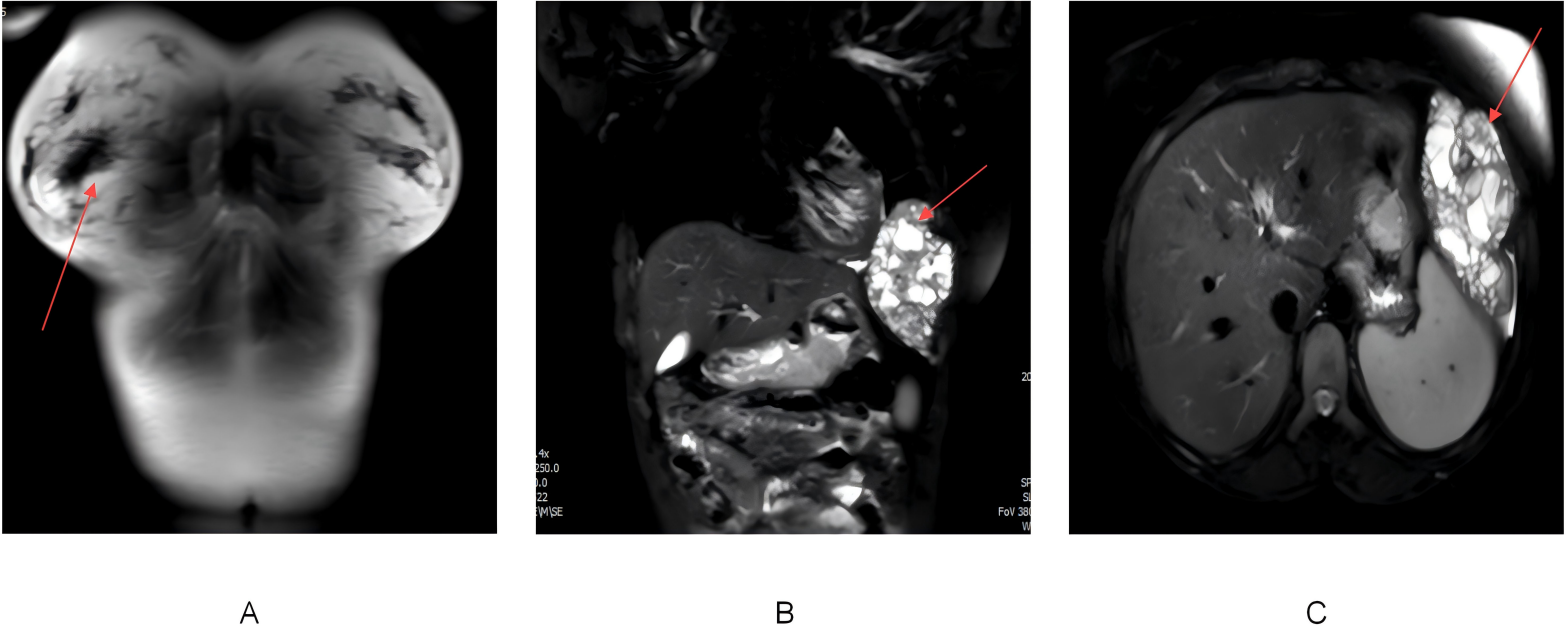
(A) MRI of the back and chest soft tissues showing expansile destruction of the left 6th rib, with an abnormal soft tissue mass in the adjacent pleural cavity. The mass appears heterogeneous on T1WI and T2-fat suppressed sequences. The red arrow marks the location of rib destruction and adjacent soft tissue mass. (B) Coronal MRI image showing a large soft tissue mass adjacent to the rib, with cystic degeneration and necrosis. The red arrow circles the cystic and necrotic area within the soft tissue mass. (C) Axial MRI image demonstrating the lesion compressing adjacent structures, including the left lung, diaphragm, and spleen. The red arrow points to the region of compression caused by the lesion.
